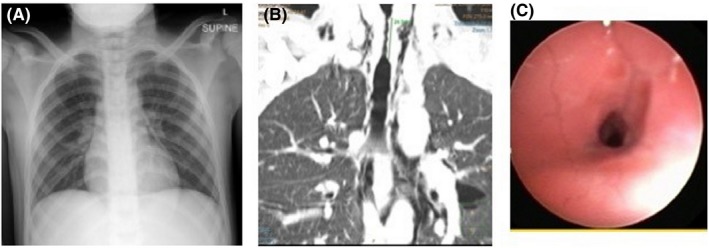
A, Chest X‐ray demonstrating narrowing of the proximal trachea and pneumomediastinum. B, Coronal chest CT‐scan reconstruction: Extensive narrowing of the proximal trachea, starting just below the subglottic area. Air is visible in the mediastinum. C, bronchoscopy picture: severe narrowing of the trachea just below the subglottic area
